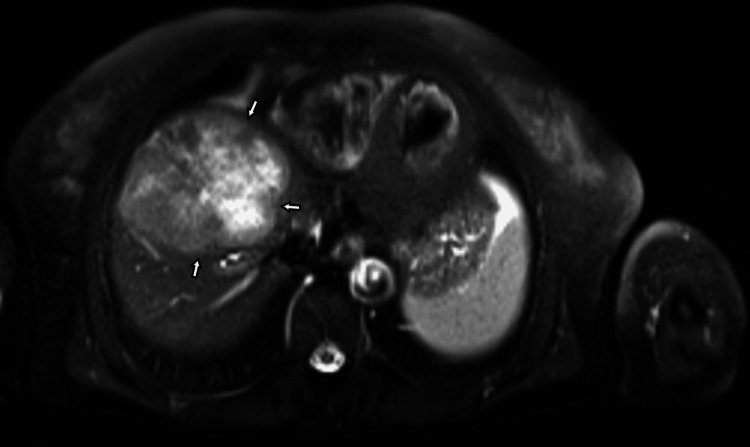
Abdominal and pelvic MRI showing a large hepatic nodule MRI: magnetic resonance imaging
